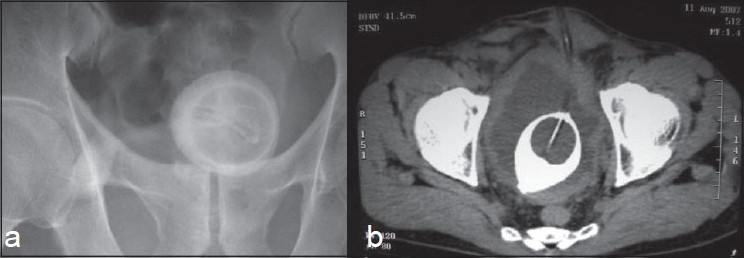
(a) X-ray pelvis shows a radiolucent core surrounded by calcification: “Egg Shell” calcification, (b) CT scan shows a calculus around the Foley balloon catheter
